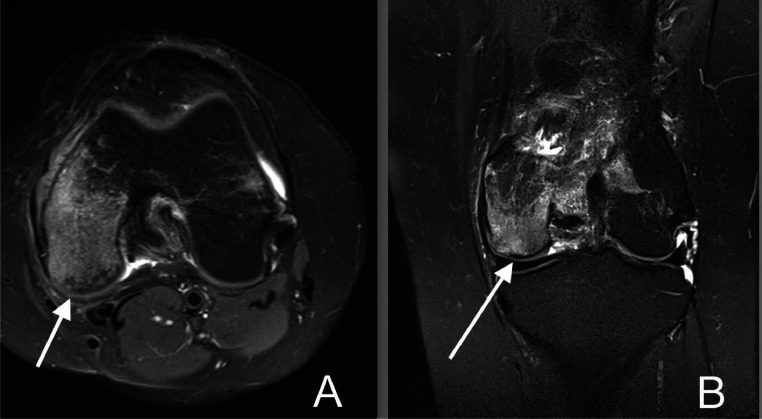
Axial (A) and coronal (B) PD fluid sensitive MRI sequences. There is indentation of the articular cartilage at the medial femoral condyle with irregularity at the subchondral bone plate, in keeping with chondral injury. There is intense oedema at the medial femoral condyle which appears out of proportion to the degree of chondral defect. Findings are suggestive of subchondral insufficiency fracture.
